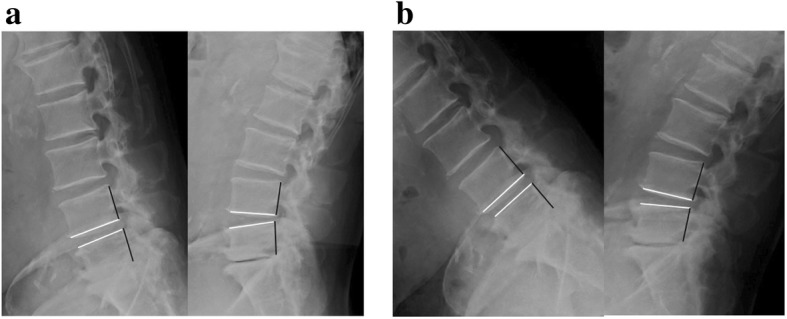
X-ray lateral image at flexion and extension of a representative patient who underwent L4–5 ULBD. a Before surgery. b Two years after surgery. The white line shows the local ROM and black line shows the translation. The change of local ROM by ULBD is − 2° and the change of translation is 0 in this patient who did not show occurrence of any severe instability after surgery
